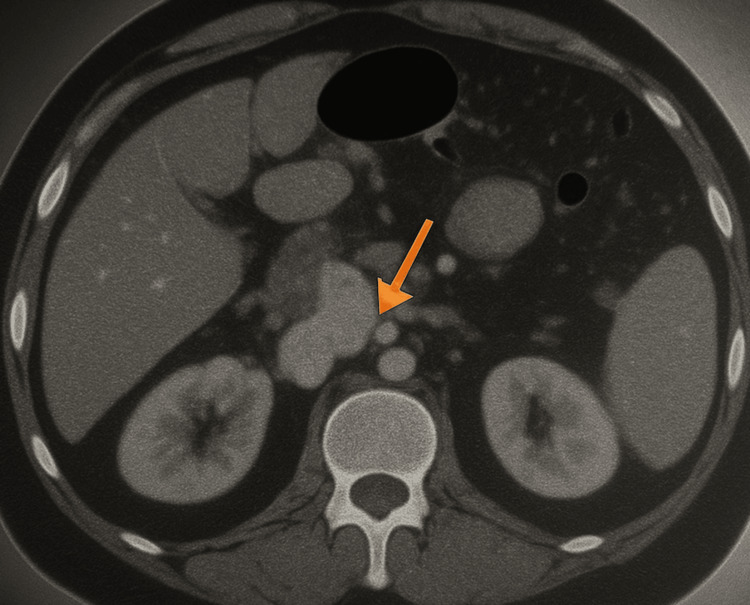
Subsequent CT abdomen showing a heterogeneous mass in the pancreas involving SMA and abutment of the aorta CT: computerized tomography; SMA: superior mesenteric artery The orange arrow indicates the pancreatic mass.
